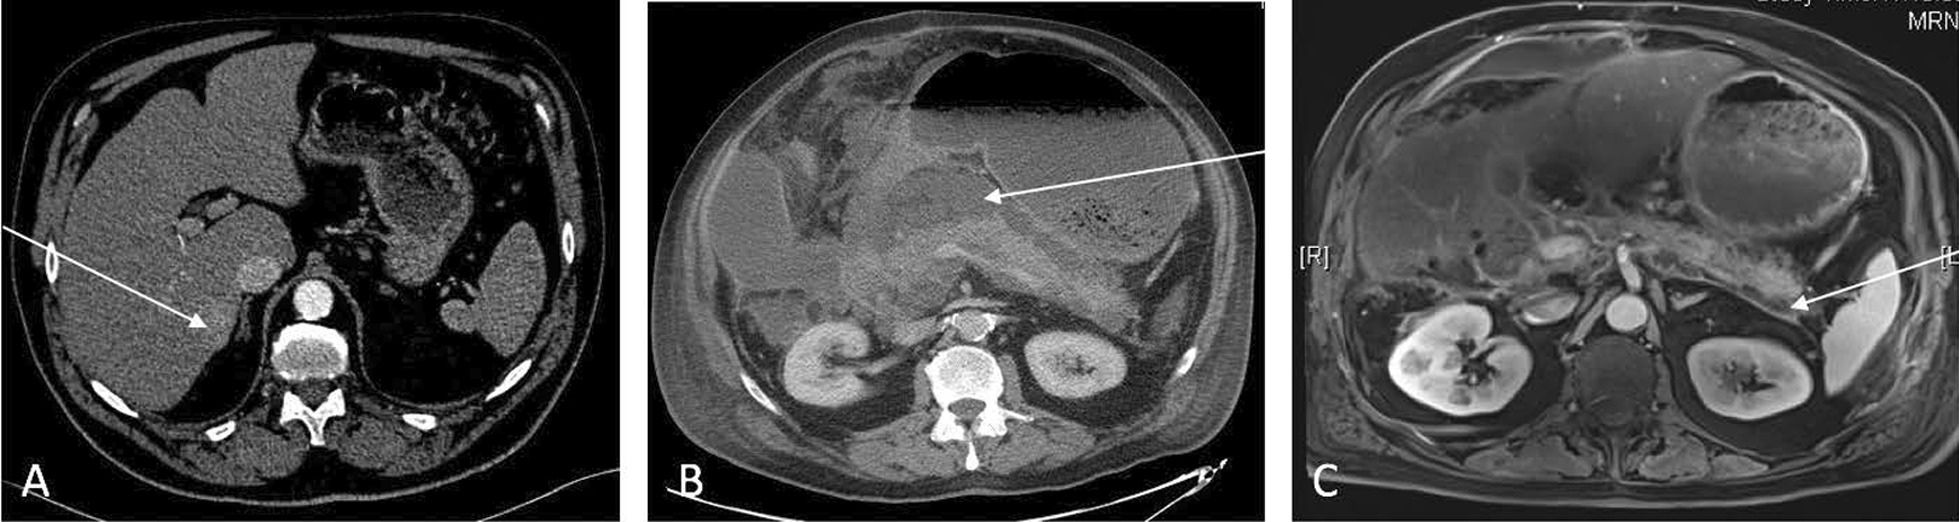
Patient C. A Preoperative CT showing HCC in segment VI. B Postoperative CT scan at day 14 showing acute pancreatitis with abdominal collections (white arrow). C MRI, T1-weight, at 1 month after acute pancreatitis showing regression of the abdominal collections (white arrow)
